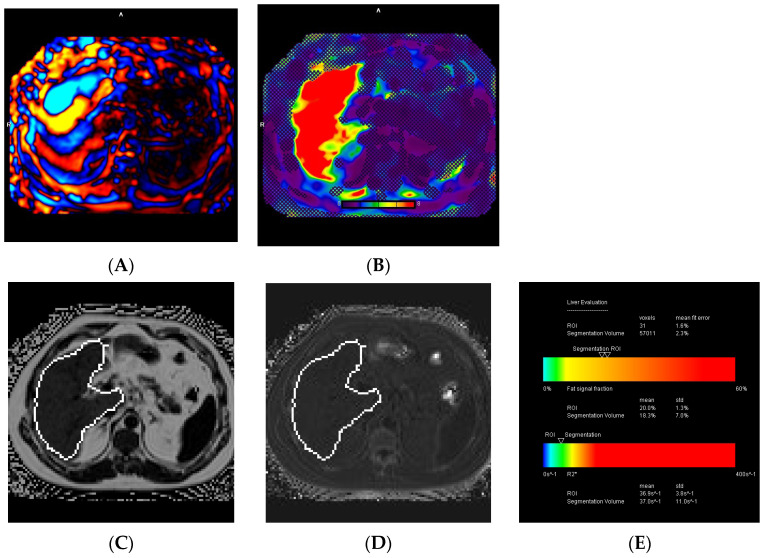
A 53-year-old male with MASLD. Wave (A) and elastogram (B) MRE images show a cirrhotic liver with a stiffness of 8.0 kPa. MRI-PDFF map (C), R2* map (D), and the liver evaluation table (E) demonstrate moderate steatosis (18.3%) and a normal liver iron (R2* of 37 s^−1^ and a LIC of 1.2 mg/g) based on segmentation.
